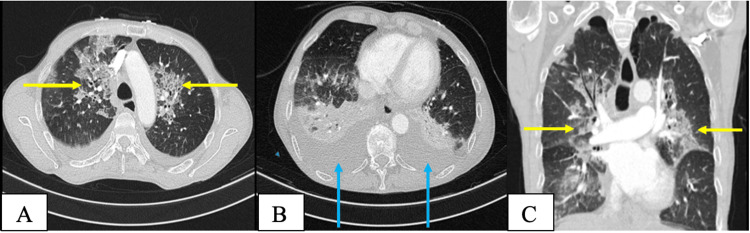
Computed tomography of the chest. A & B-Axial views, C- Coronal view. Yellow arrows indicate areas of atelectasis, blue arrows indicate bilateral pleural effusion.
